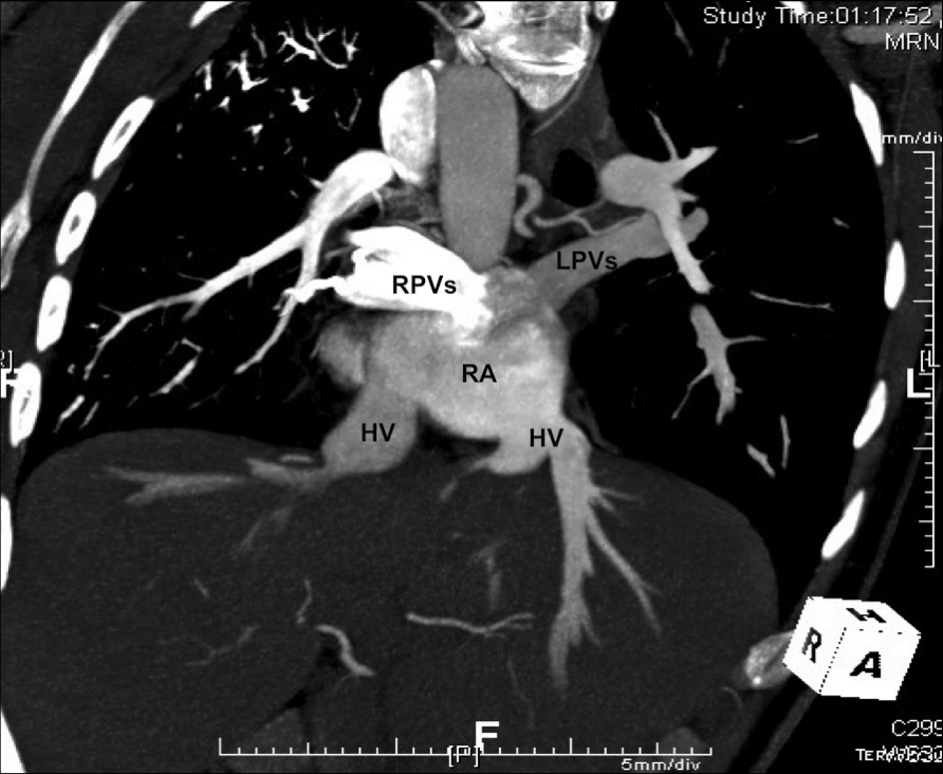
CT angiography scan of a patient with left isomerism and interruption of the inferior vena cava, showing the presence of separate hepatic veins draining into the right atrium, and the presence of total anomalous pulmonary venous connection with “polarized” connection of the pulmonary veins to the right atrium, the right connected to the right side and the left to the left side. HV, hepatic vein; LPVs, left pulmonary veins; RA, right atrium; RPVs, right pulmonary veins.
